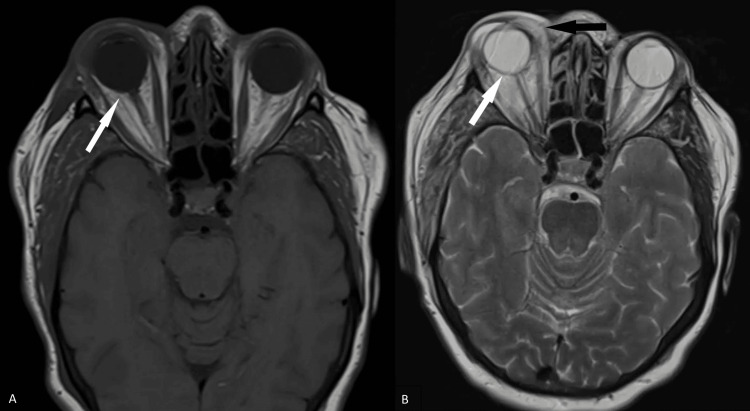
MRI images of the brain showing findings of right orbital cellulitis and increased intraorbital pressure. Axial T1-weighted and T2-weighted sequences demonstrate tenting of the right posterior globe (white arrows, A and B) suggestive of increased right intraorbital pressure. The axial T2-weighted sequence also shows increased T2 signal intensity throughout the extraconal palpebrae soft tissues spanning from the medial to lateral epicanthal folds consistent with right orbital cellulitis (black arrow, B).
